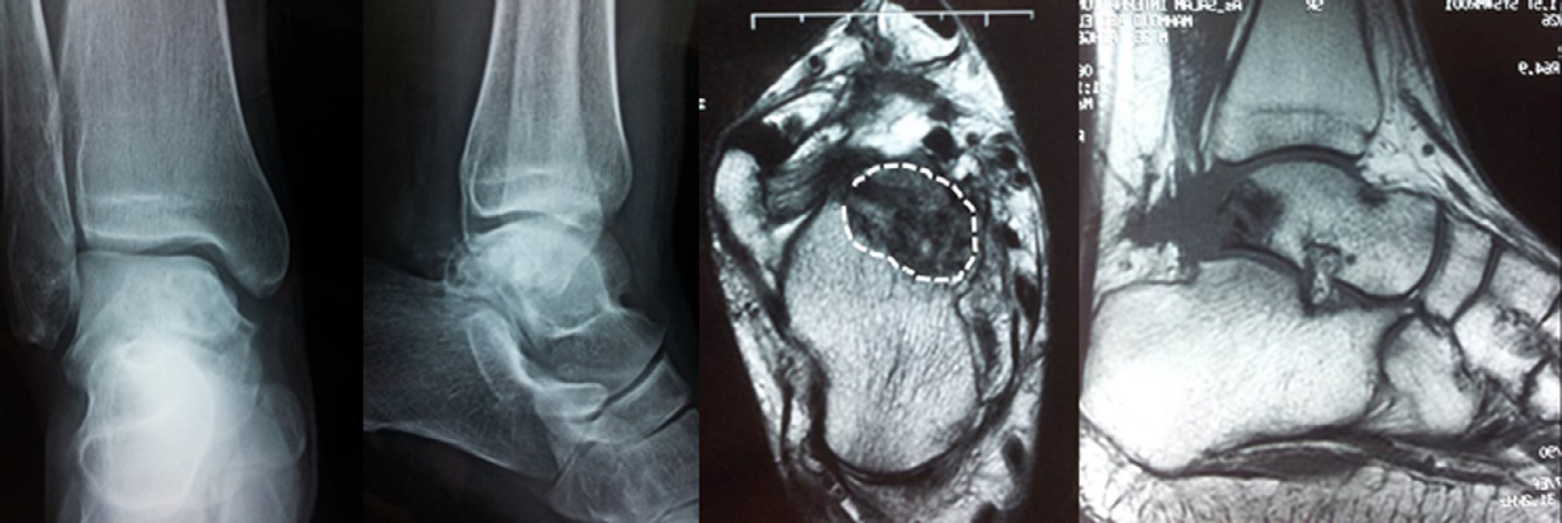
Radiological evaluation of the cyst after 2 years by plain X ray and MRI showing complete healing of the cyst with no recurrence.
